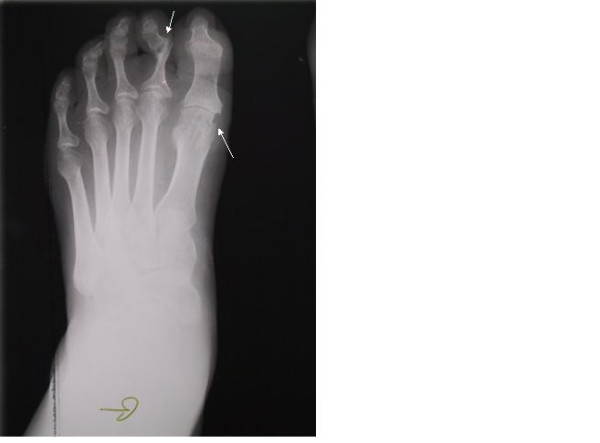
X-ray of a left foot. Erosive lesion in the first metatarso-phalangeal joint, and subluxation and erosion of the second proximal inter phalangeal joint similar to psoriatic arthritis.
